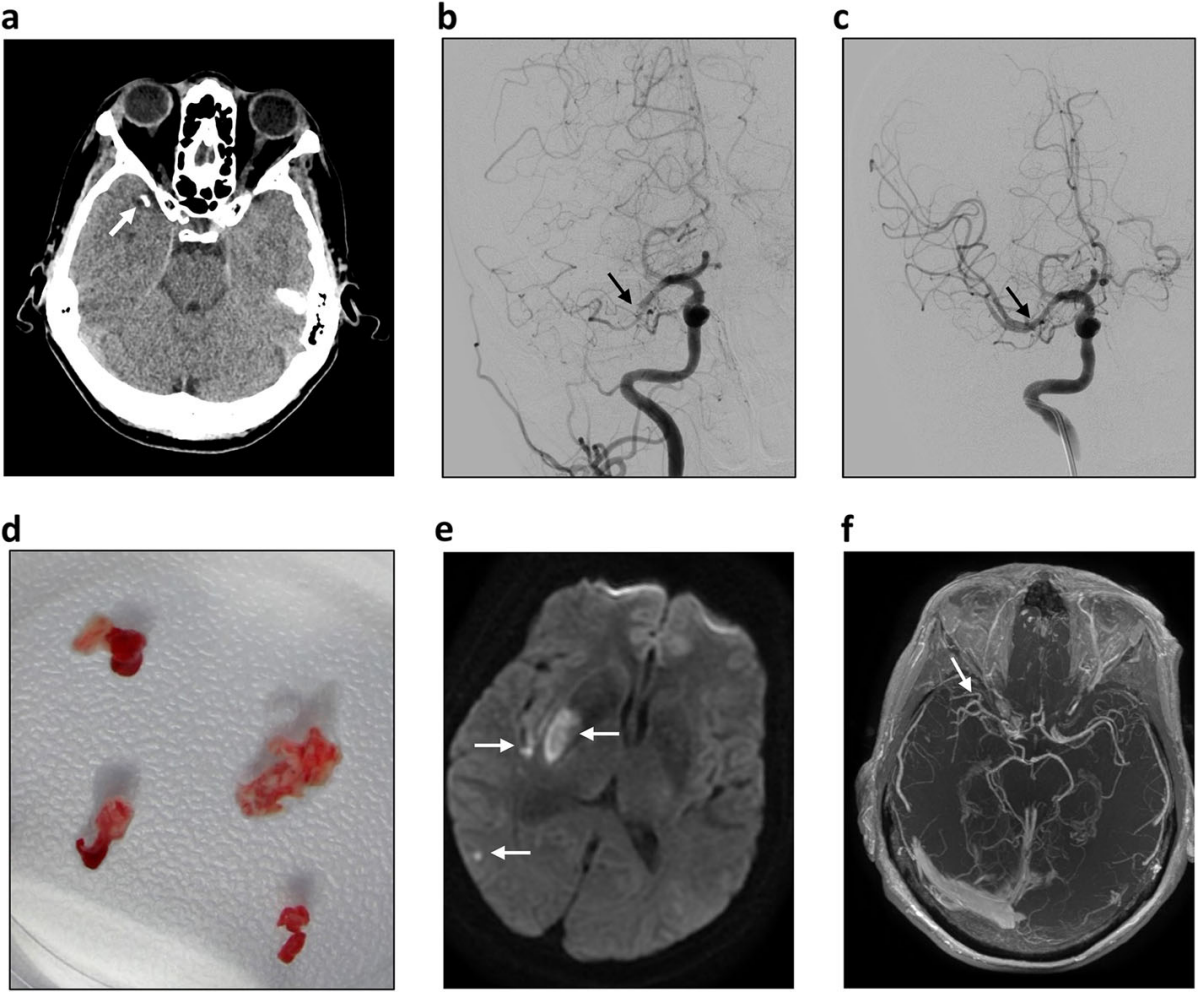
Radiological imaging of the patient. A non-contrast CT scan of the head revealed a right hyperdense middle cerebral artery sign (average 138 HU, white arrow), with already infarction in the basal ganglia (not shown) in the right hemisphere but no signs of intracranial hemorrhage (a). On DSA, initial runs confirmed a complete occlusion of the distal M1-segment of the right middle cerebral artery (black arrow) with good pial collateralization (b). Final angiographic result after the 11th thrombectomy attempt showing a complete recanalization of the right middle cerebral artery (c, black arrow). The thrombus appeared hard and white macroscopically (d). DWI-MRI demonstrated restricted diffusion in the right basal ganglia and several smaller cortical and subcortical lesions 24 h after the endovascular treatment (e, white arrows) and MRA showed recanalization of the previously occluded segment of the right middle cerebral artery (f, white arrow)
